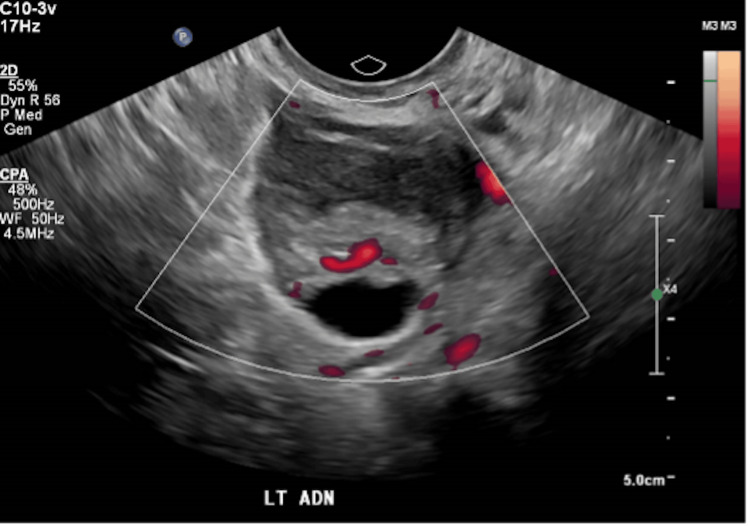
Ultrasound pelvis showed a 3.7 x 3.0 x 2.8 cm tubo-ovarian complex, with convoluted tubular cystic structure with low-level echoes and a segment with a thick vascular wall.
